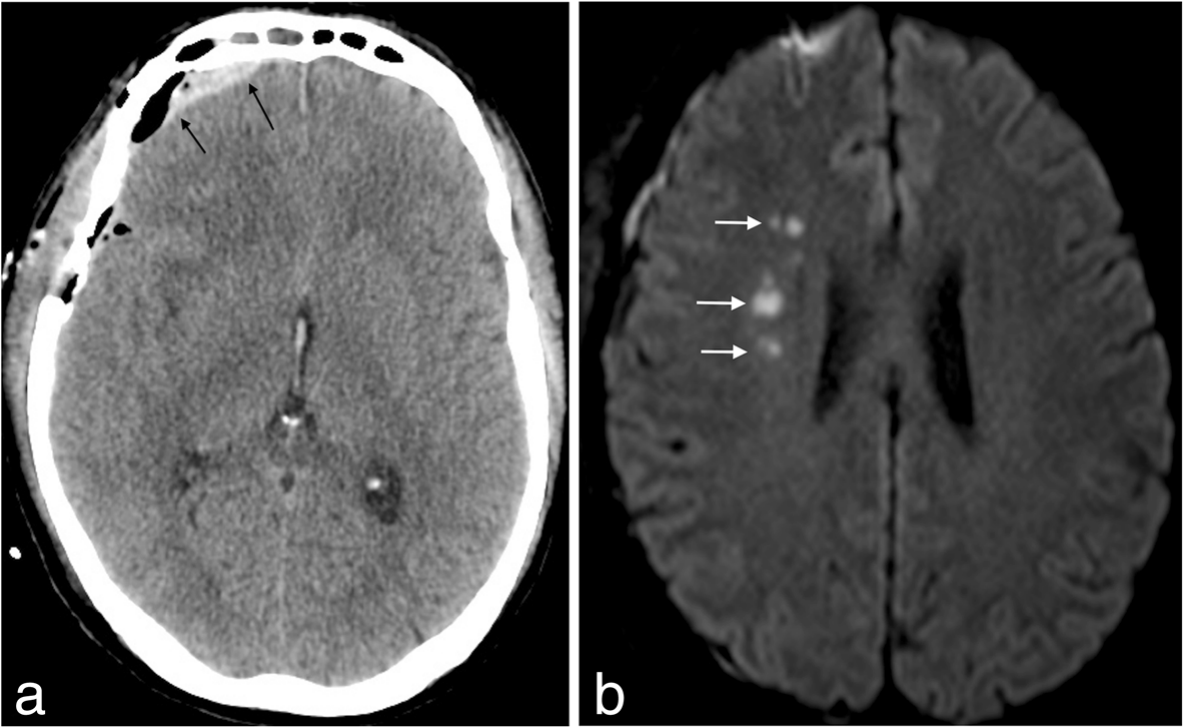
Post-surgical CT visualization of a right frontal heterogeneous collection (2a, left). MRI showed no empyema but ischemic lesions of the right deep frontal white matter (2b, right)
